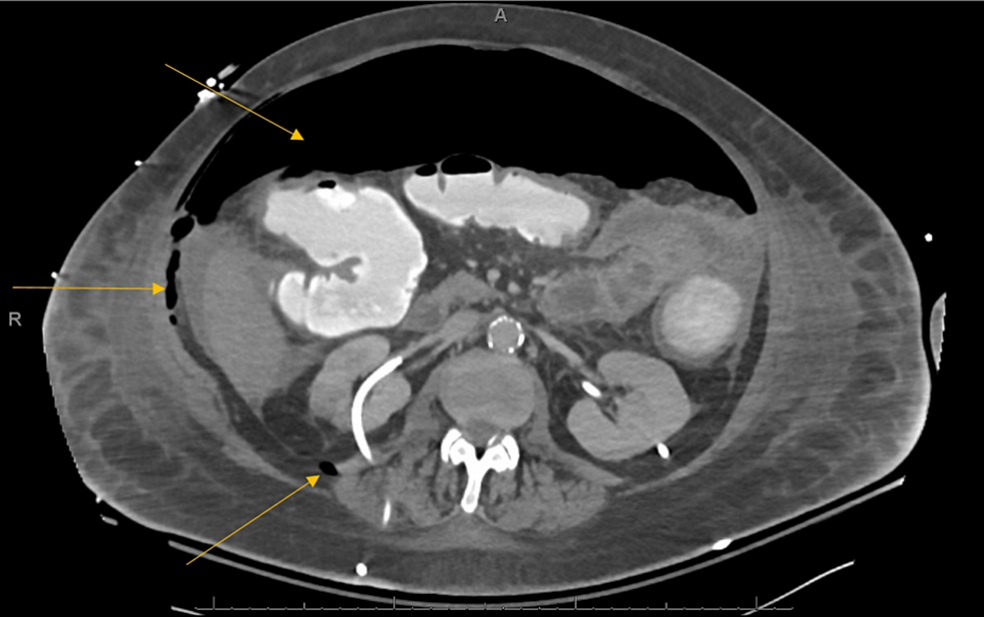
Diffuse-free intraperitoneal and retroperitoneal (arrows) air consistent with viscous perforation on CT scan.
